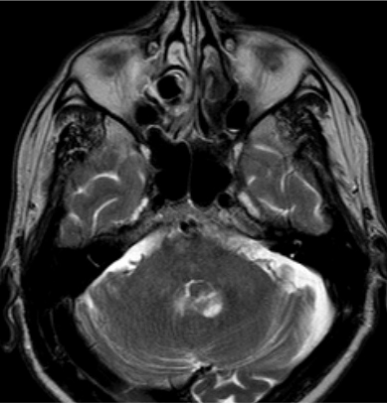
Axial T2 weighted magnetic resonance image of 4th ventricle choroid plexus carcinoma
